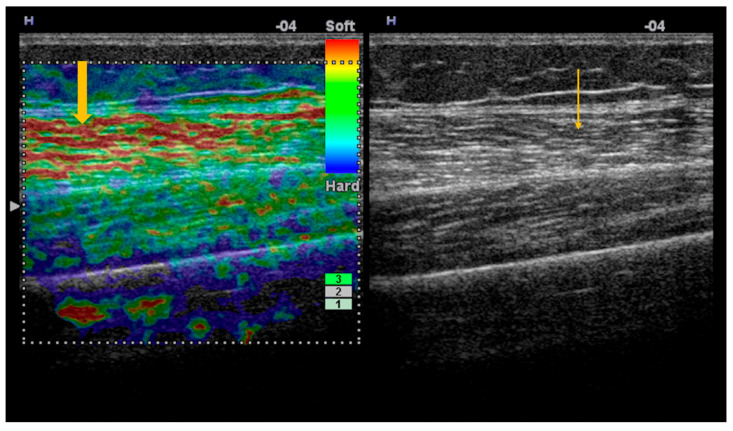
Strain elastography and corresponding gray-scale imaging of myositis with fatty infiltration. Strain elastography of the thigh skeletal muscle demonstrates reduced tissue stiffness, as indicated by red color coding on the elastography map (thick arrow), indicating softening of the affected muscle. The corresponding gray-scale ultrasound image shows hyperechoic muscle texture (thin arrow), consistent with fatty infiltration.
